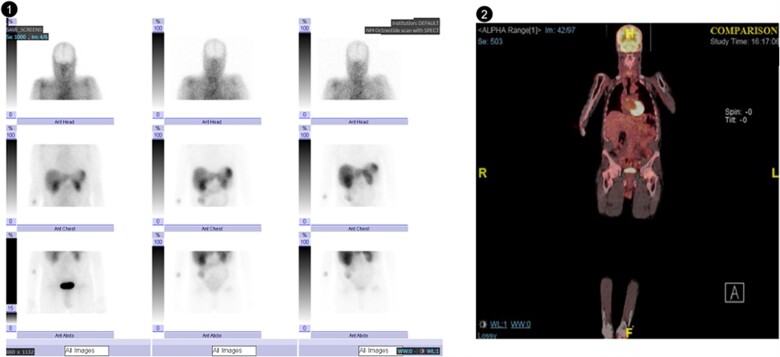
(1) Octreotide scans with single-photon emission computed tomography showing physiological uptake in the liver, spleen, kidneys and large bowel. The focal area of uptake related to the right area corresponds to the isotope injection site. No focal abnormal area of uptake was demonstrated. (2) Fluorodeoxyglucose-18 (FDG) positron emission tomography (PET) scan showing no abnormal activity.
